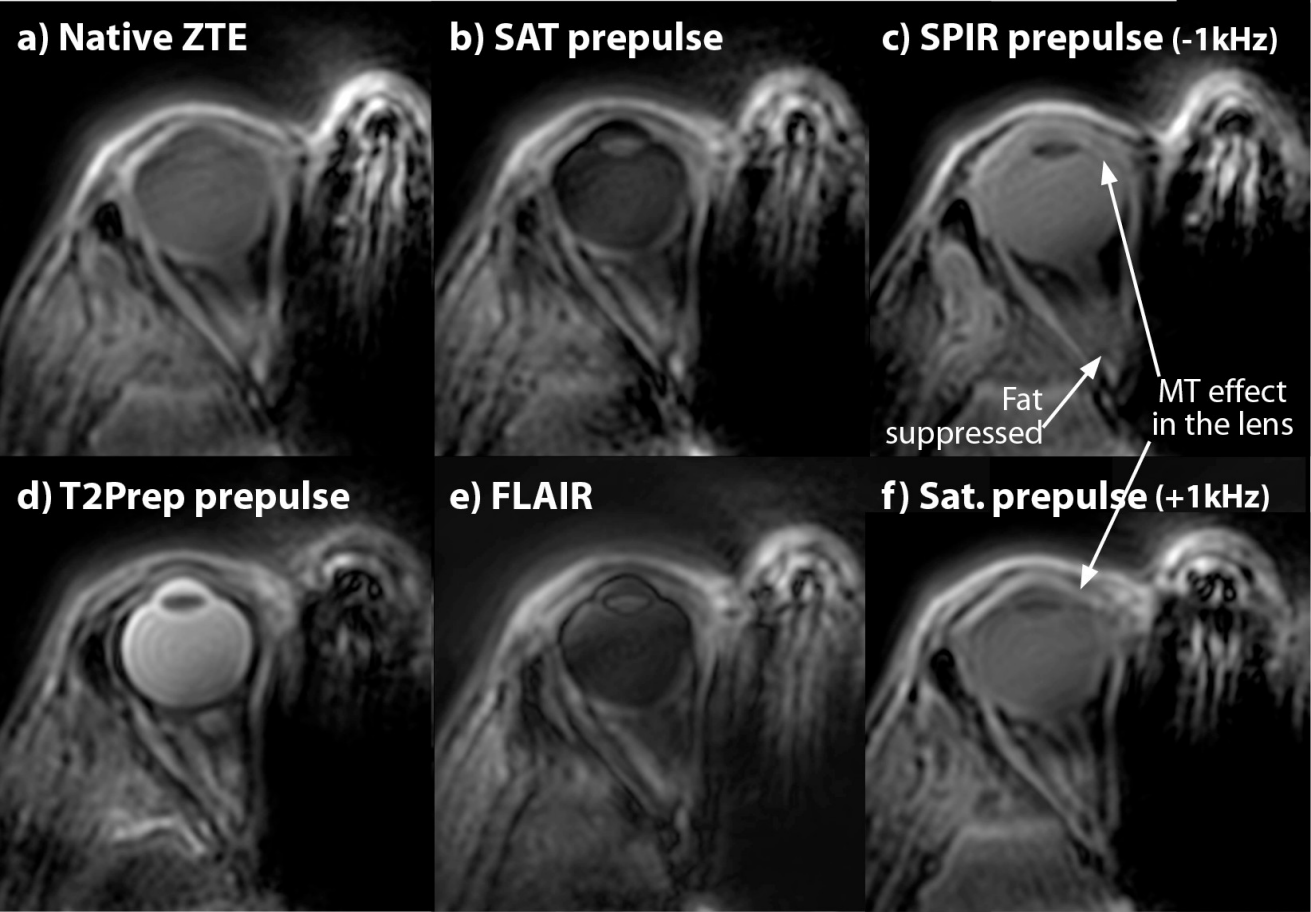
Magnetization-prepared eye ZTE imaging. One selected reformatted slice from the 3D data sets of a healthy volunteer. (a) native ZTE. The eye and parts of the bony structures (especially in the nose area) are visible. Due to the short TE and the low flip angle, the overall contrast is low. (b) A SAT pre-pulse inverts the contrast between eyeball and lens. (c) Fat suppressed (SPIR) ZTE showing improved contrast in the eye and around the nerve and the lens is now visible. (d) T_2_-prepared shows the highest contrast of all sequences. (e) FLAIR-ZTE contrast has low signal from the vitreous body and high signal from the lens and sclera. (f) ZTE image like in (c) but applying the fat saturation RF pulse symmetrically on the other side of the water resonance. This shows no fat suppression but slight contrast in the lens, indicating MTC effect caused by saturating bound water protons.
